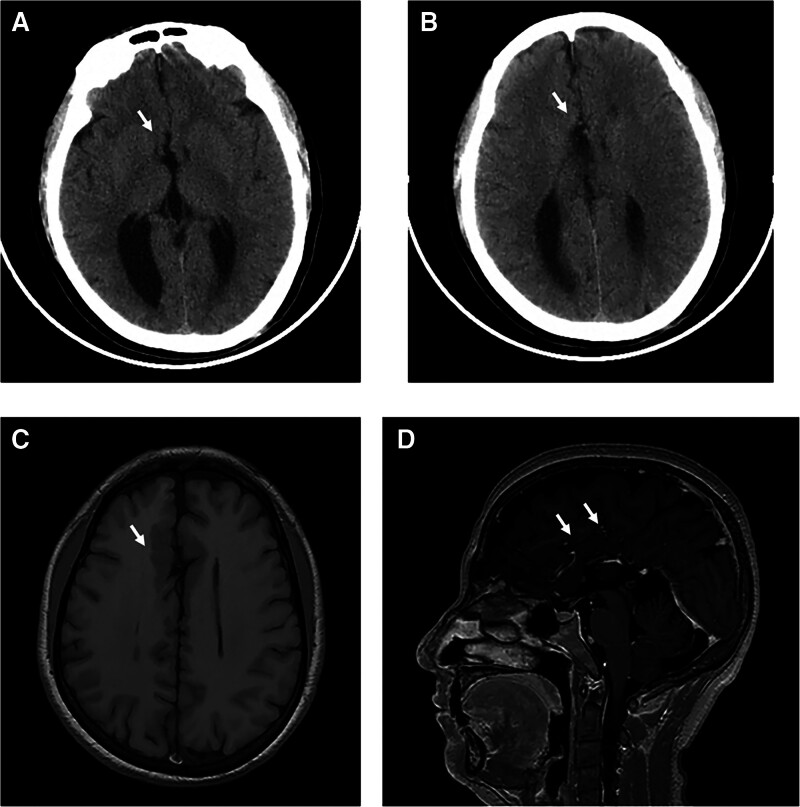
Corpus callosum agenesis. (A, B) Axial noncontrast CT showed corpus callosum agenesis (white arrowheads). Axial T1-weighted image without contrast (C) and sagittal contrast-enhanced T1-weighted image (D) confirmed the agenesis of corpus callosum with gray matter heterotopia (white arrowheads). CT = computed tomography.
